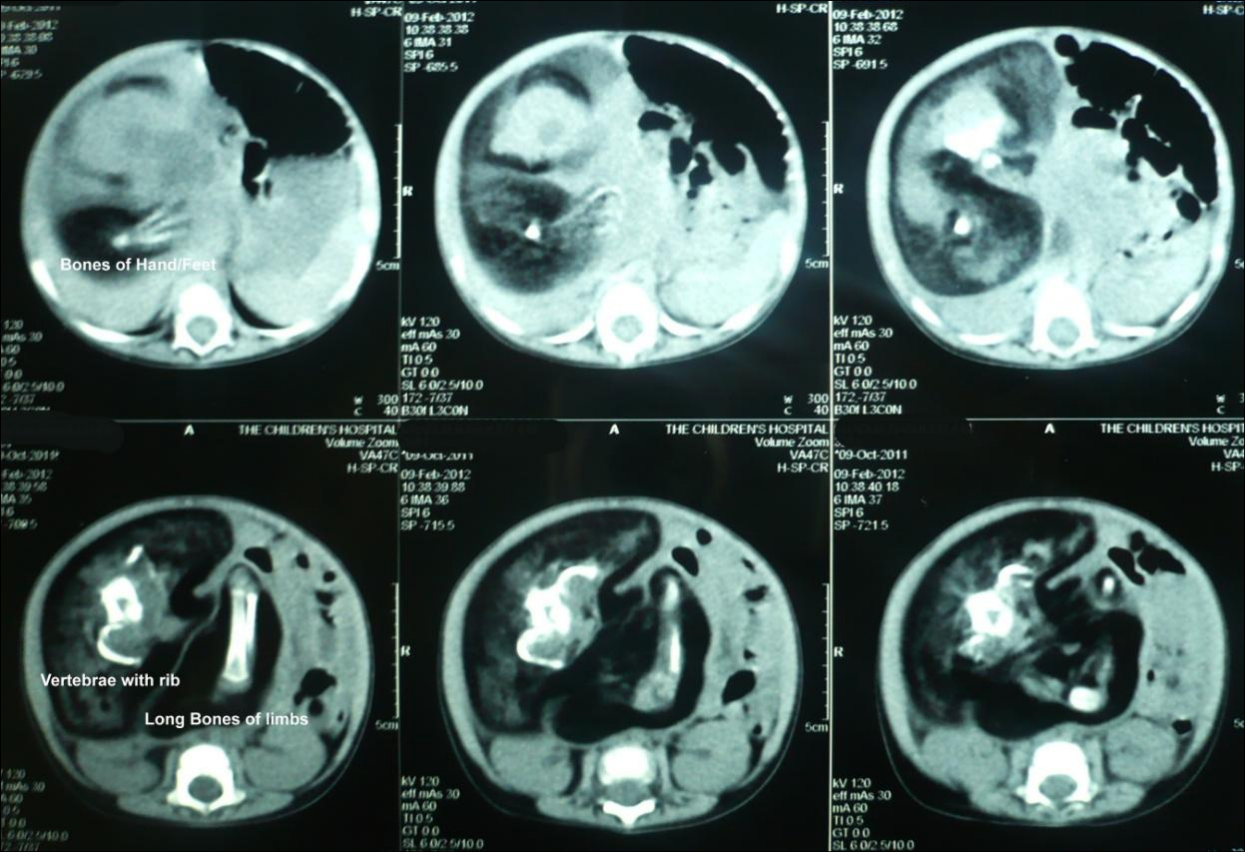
Figure 1: CT scan showing various kind of bones in FIF.
